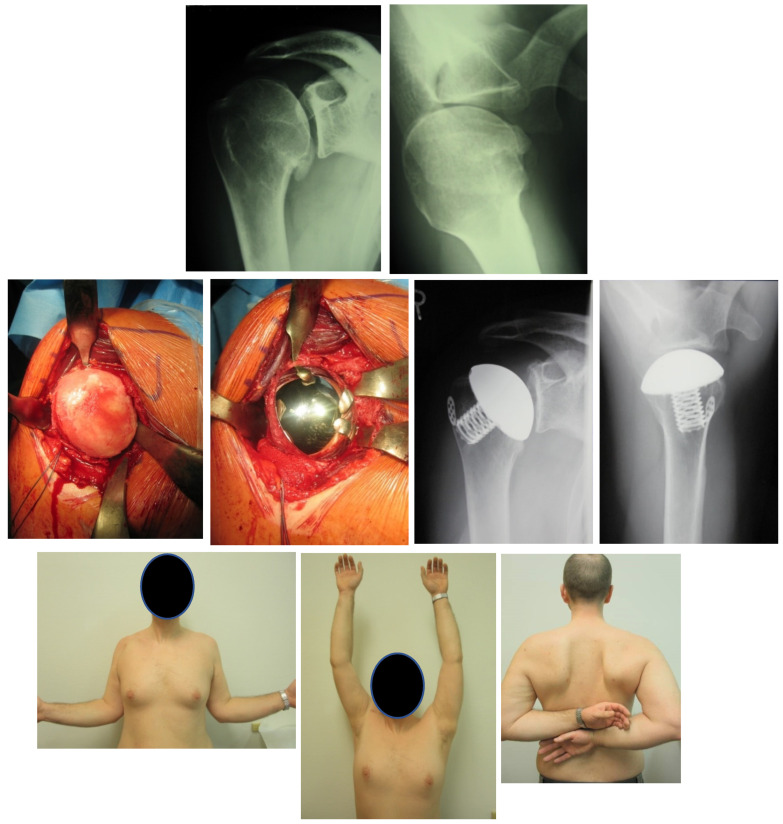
43 year-old patient treated with hemiprosthesis after capsulorrhaphy arthropathy subsequent to open instability repair. First row: preoperative radiographs. Second row: intraoperative pictures of hemiprosthesis implant and postoperative radiographs. Third row: Physical examination and shoulder function at final follow-up.
